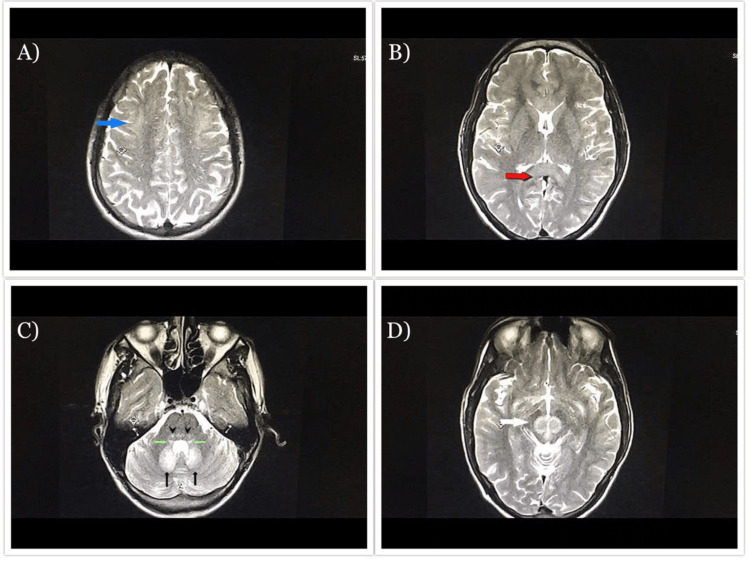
0.4 Tesla MRI and axial T2WI demonstrate a hyperintense signal. (A) Subcortical white matter (blue arrow). (B) Splenium (thick red arrow). (C) Cerebellar dentate nuclei (thin black arrows), vestibular (green arrows), and a superior olivary nuclei lesion of caudal pons (arrowheads). (D) Midbrain involving red nuclei and tegmentum (white arrow). MRI: magnetic resonance imaging; T2WI: T2-weighted image
